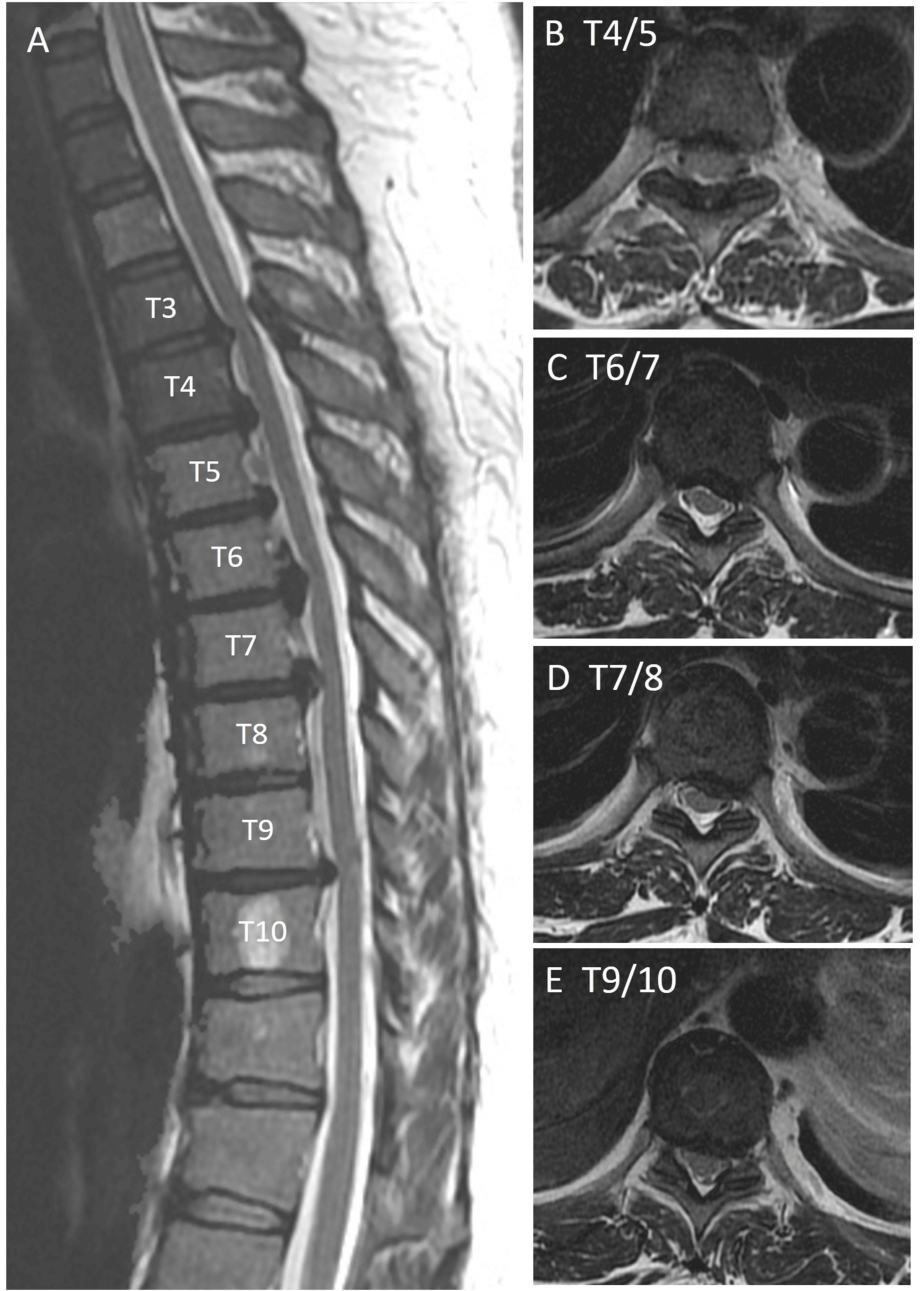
Preoperative T2-weighted MRI of the thoracic spine (A) Sagittal view. Segmental ossification of the posterior longitudinal ligament causing moderate spinal cord compression. (B) T4/5 level stenosis. (C) T6/7 level stenosis. (D) T7/8 level stenosis. (E) T9/10 level stenosis.
